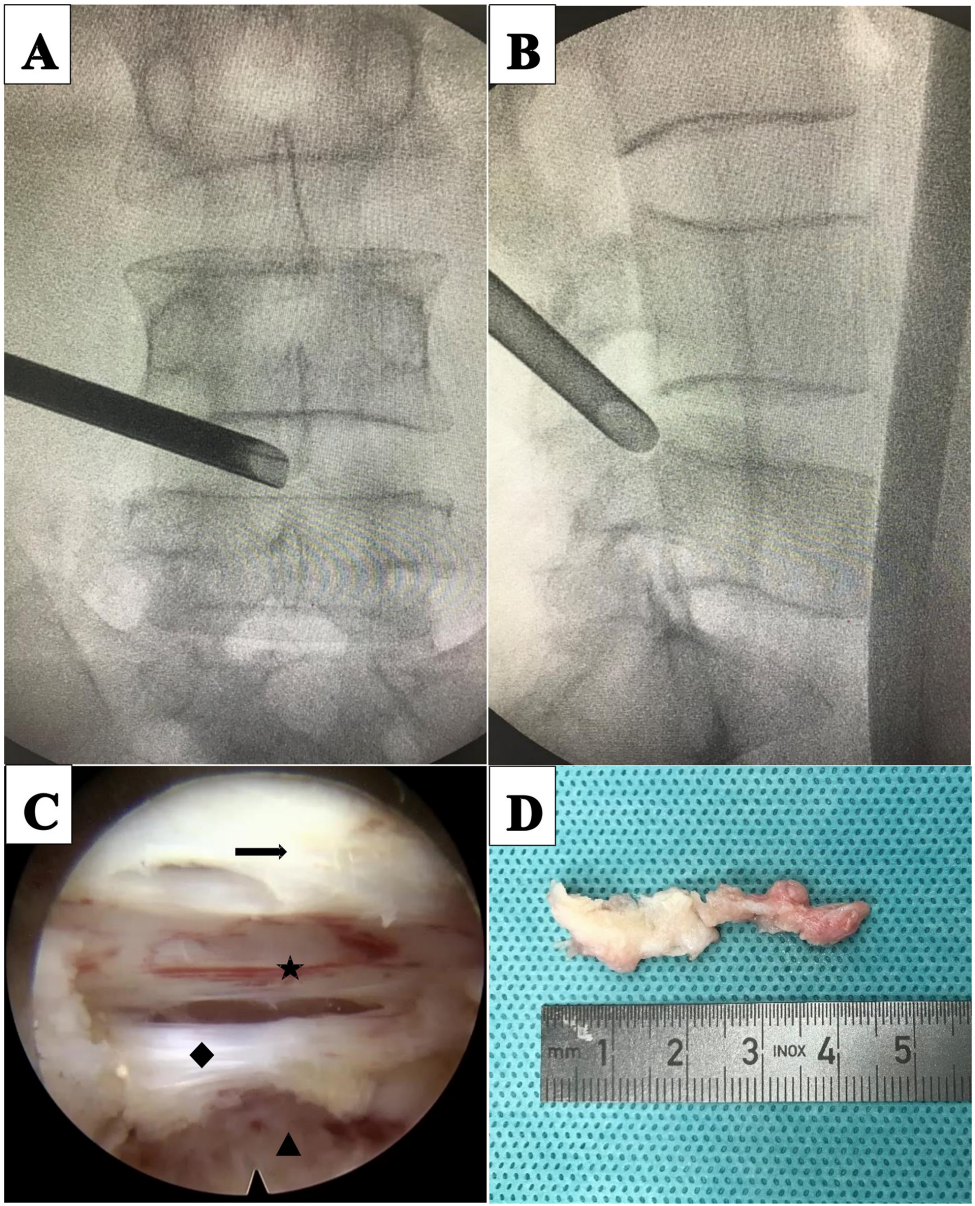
Percutaneous endoscopic transforaminal discectomy (PETD) for a 64-year-old male patient with L4–L5 disc herniation. (A) Intraoperative anteroposterior x-ray shows the position of the working cannula. (B) Intraoperative lateral x-rays shows the working cannula reaching the ventral side of the dural sac. (C) Intraoperative images; (arrow) ligamentum flavum, (star) dural sac, (diamond) posterior longitudinal ligament, (triangle) disc. (D) Disc pulposus.
